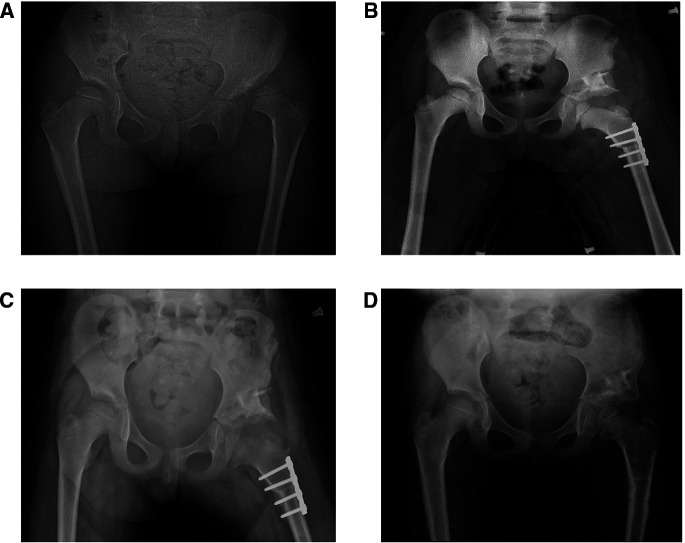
A girl, aged 6 years and 8 months, with Tönnis II dislocation of her left hip, underwent a Pemberton osteotomy combined with femoral shortening osteotomy. (A) Preoperative hip anteroposterior (AP) radiograph: left hip dislocation. (B) AP radiograph obtained three days after the Pemberton osteotomy combined with femoral shortening osteotomy; the length of the femoral osteotomy is 2 cm. (C) AP radiograph obtained 1.5 months after the Pemberton osteotomy combined with femoral shortening osteotomy. (D) AP radiograph obtained 12 months after the Pemberton osteotomy combined with femoral shortening osteotomy and 2 months after removal of internal fixation.
